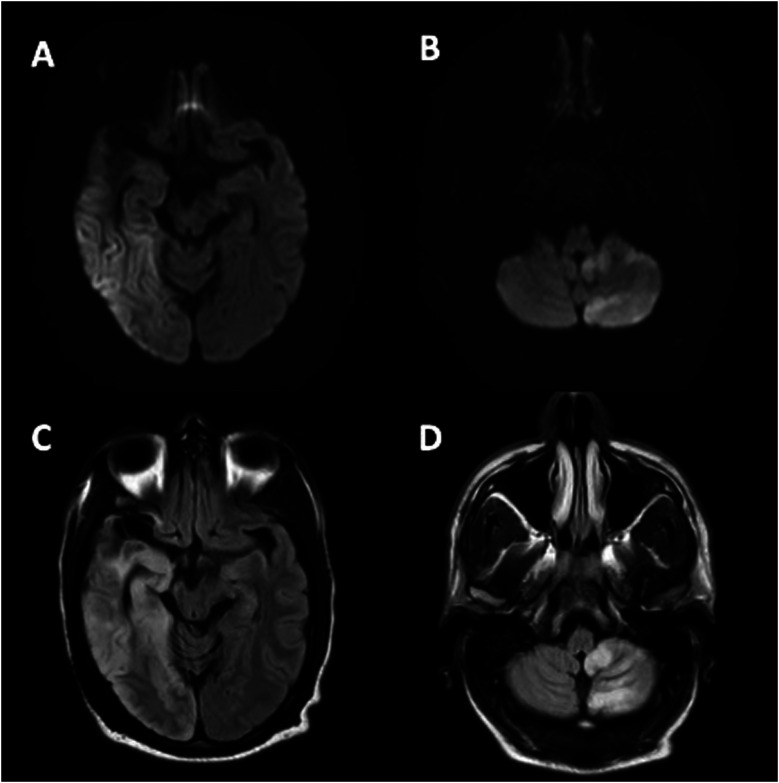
Brain MRI at presentation*: Restricted diffusion (A), (B) with T2 FLAIR hyperintensities (C), (D) in the right temporoparietal cortex (A), (C) and left cerebellar hemisphere (B), (D).
